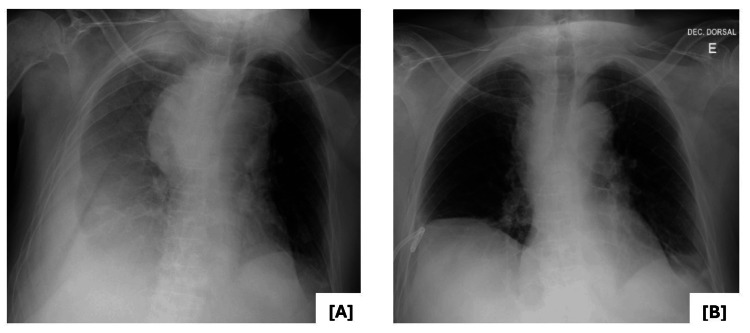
Chest X-ray A) before and B) after chest tube placement, completion of corticosteroid therapy, and radiotherapy sessions. Actual scale unavailable due to image export limitations.
